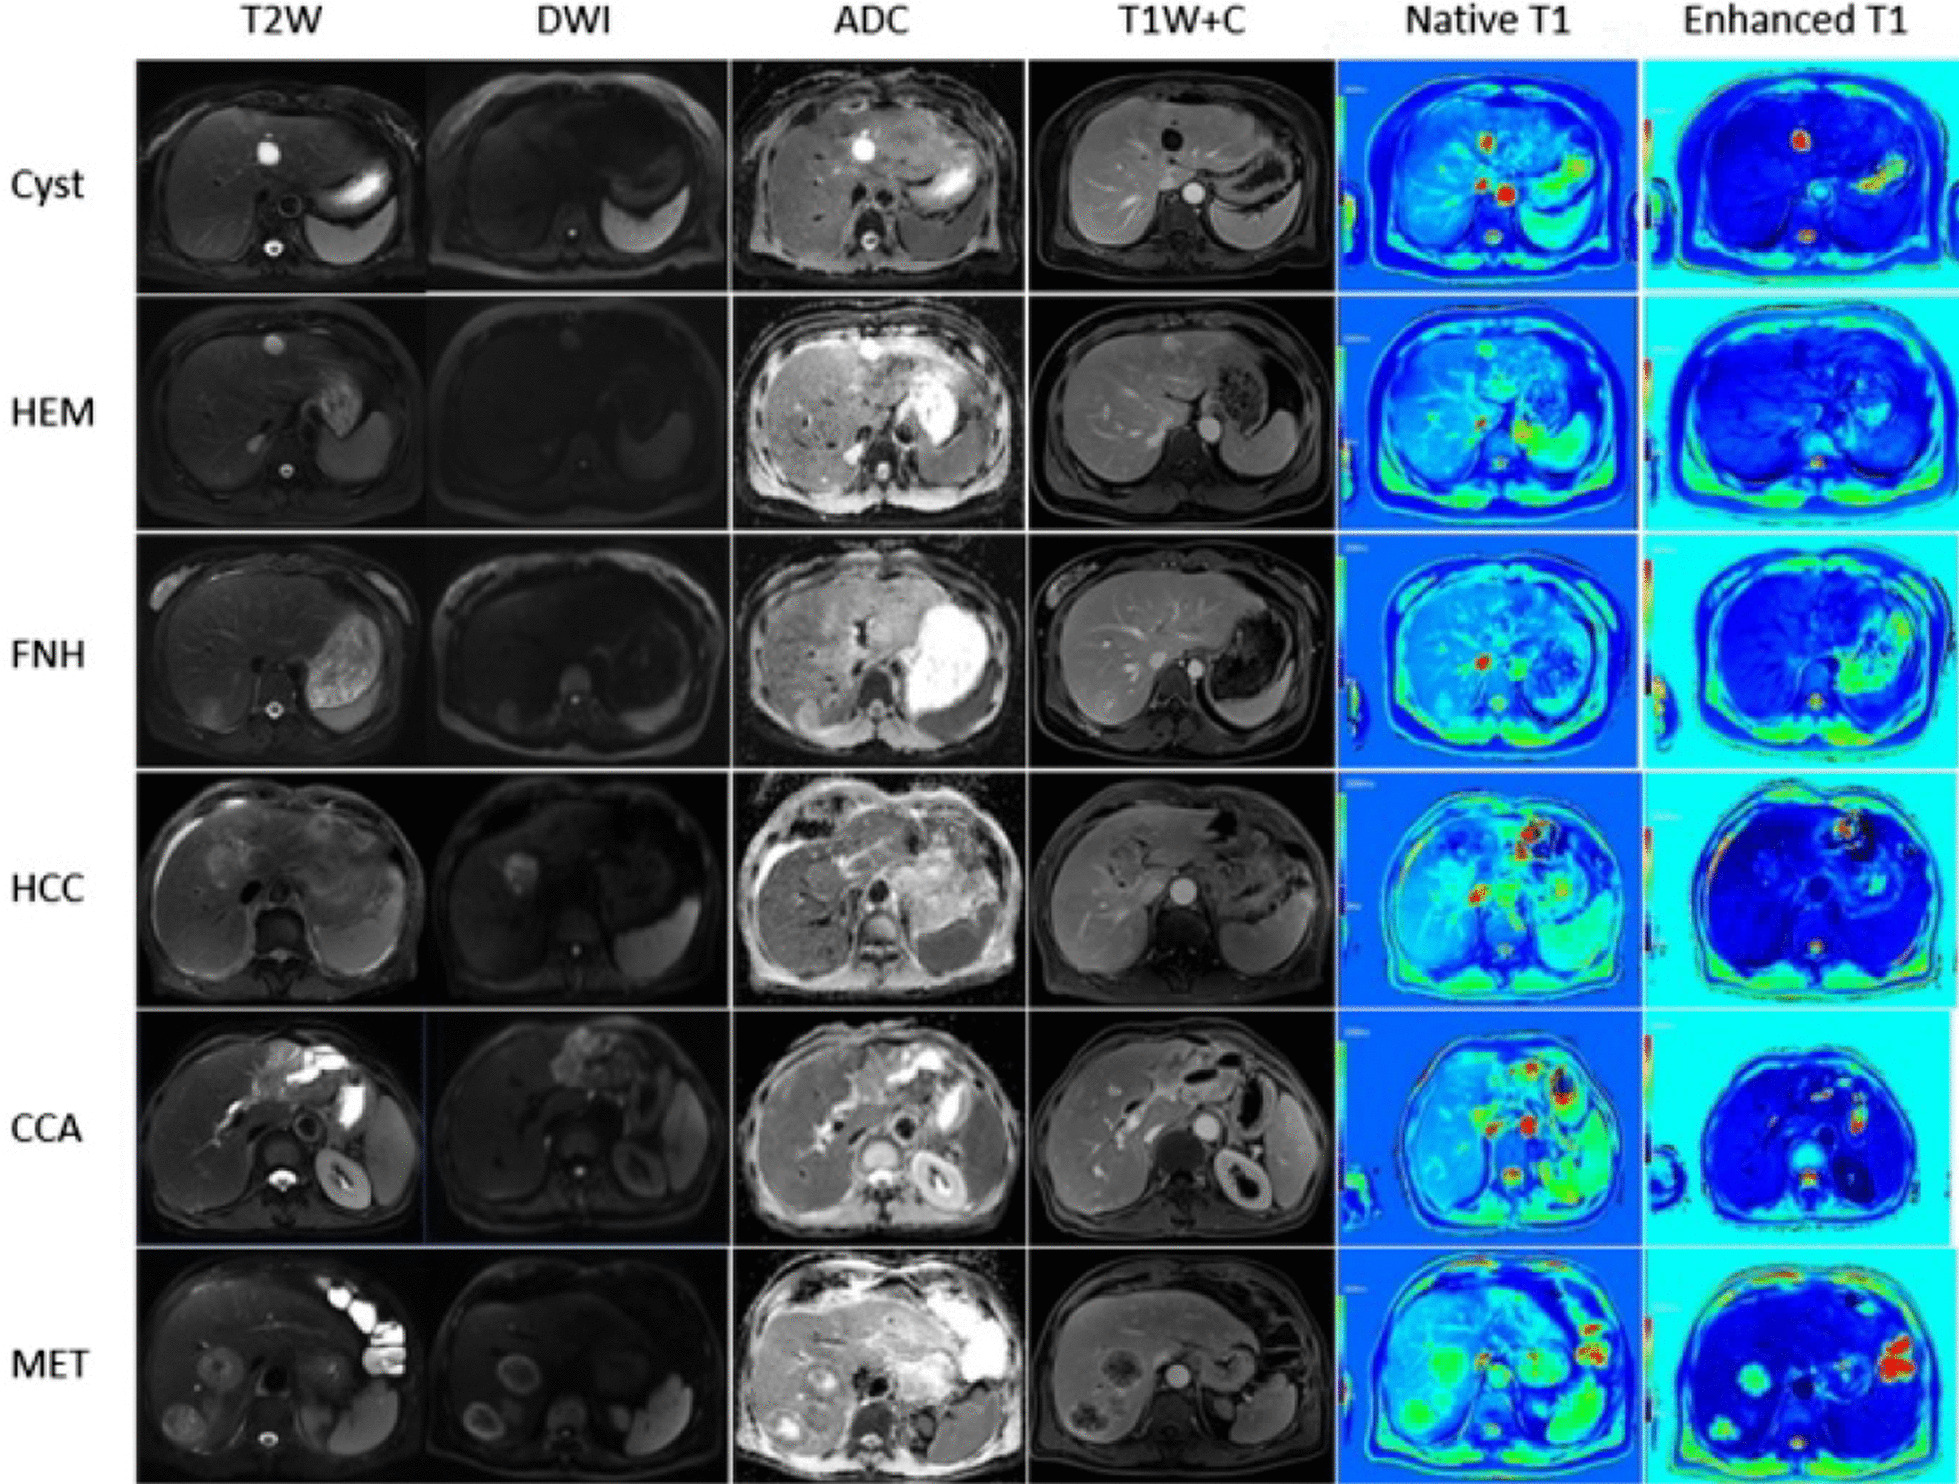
Representative images of patients with FLLs. Column 1: T2WI, Column 2: DWI (b = 1200 s/mm^2^), Column 3: ADC maps, Column 4: contrast enhanced T1W acquired 2 min after the injection of contrast agent, Column 5: native T1 mapping image, Column 6: enhanced T1 mapping images. Row 1: Cyst, Row 2: HEM, Row 3: FNH. Row 4: HCC. Row 5: CCA, Row 6: MET. HEM = haemangioma, FNH = focal nodular hyperplasia, HCC = hepatocellular carcinoma, CCA = cholangiocarcinoma, MET = hepatic metastases
